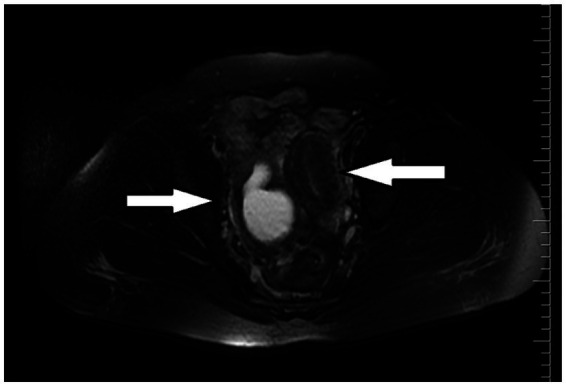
MRI: Axial T2-weighted image shows the presence of two uterine bodies (white arrows).
